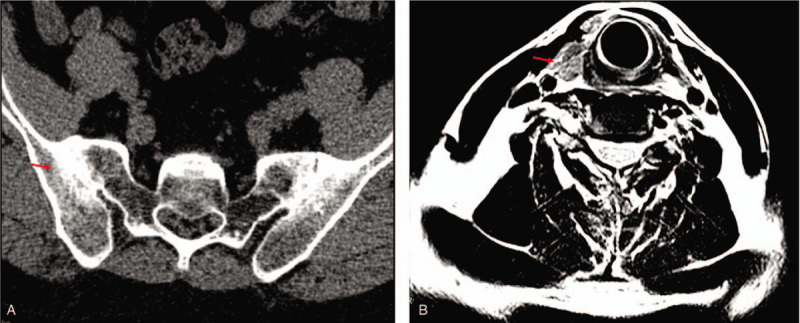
(A) Computed tomography scans of the sacroiliac joint depicted hyperosteogeny, bone destruction, joint space stenosis, and bony ankylosis. (B) Magnetic resonance imaging scans revealed subcutaneous masses on the left side of the neck.
